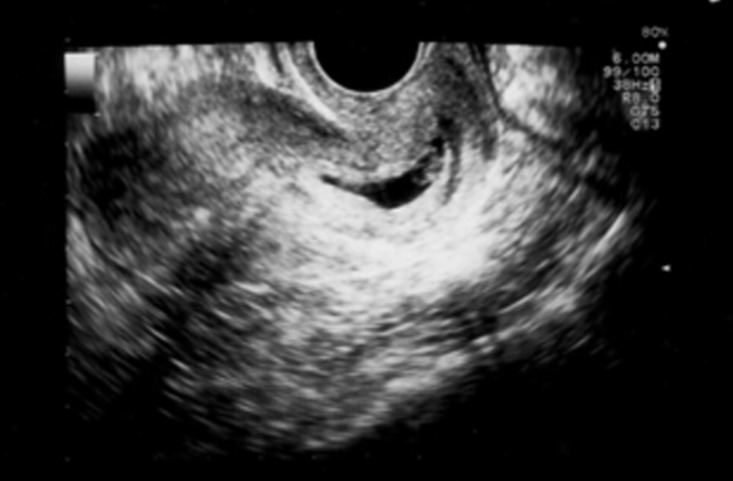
Sagittal endovaginal ultrasound of a cervical EP.
